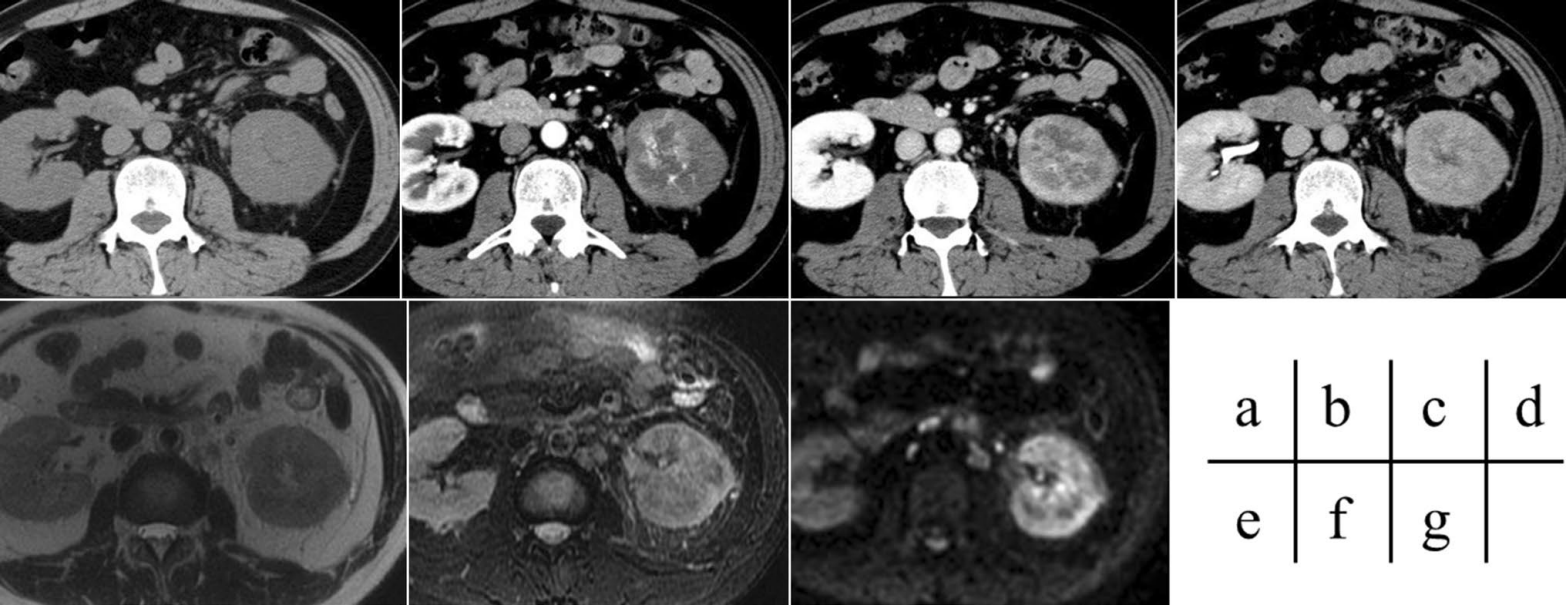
Images from a 44-year-old man with unclassified RCC. A mass lesion with invasive growth is apparent in the left kidney. The tumor appears isointense on unenhanced CT (a) and exhibits a progressive pattern on DCE imaging (b–d). The tumor also appears isointense on T1-weighted imaging (e), mildly hypointense on T2-weighted imaging (f), and hyperintense on DWI (g). No pseudocapsule is clearly identifiable. Unclassified RCC was diagnosed after surgery. The patient developed postoperative recurrence and died of cancer on Day 358
